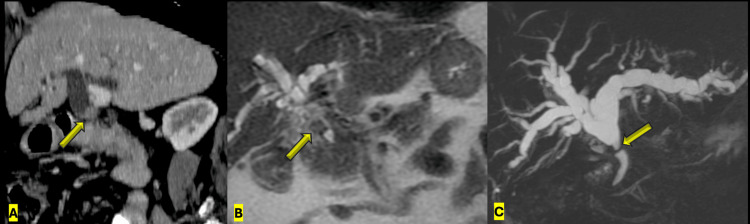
Cross-sectional imaging findings of proximal biliary obstruction A: Contrast-enhanced CT (oblique coronal reconstruction) demonstrating dilation of the common hepatic duct with an intraductal nodular soft-tissue lesion (arrow). B: Coronal T2-weighted MRI showing a hypointense nodular lesion at the confluence of the right and left hepatic ducts, with proximal intrahepatic biliary dilation and short-segment narrowing of the distal common hepatic duct. C: Oblique maximum intensity projection (MIP) reconstruction demonstrating intrahepatic ductal dilation up to the biliary confluence, short-segment occlusion, and visualization of the distal hepatocholedochus beyond the obstruction.
